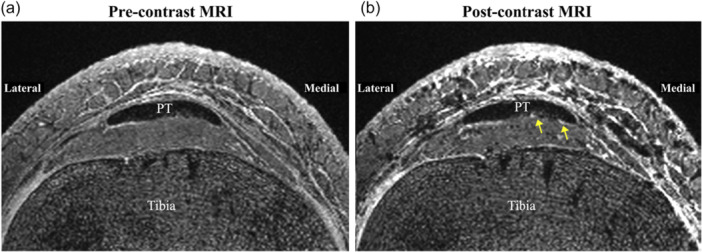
Example 7‐Tesla quantitative magnetic resonance imaging (7T‐qMRI) was acquired along axial plane. Siemens volumetric interpolated breath‐hold examination (VIBE) MRI sequence images demonstrate pre‐ (a) and post‐contrast (b) imaging of the patella tendon (PT) including increased signal at the dorsomedial border of the PT (yellow arrows).
